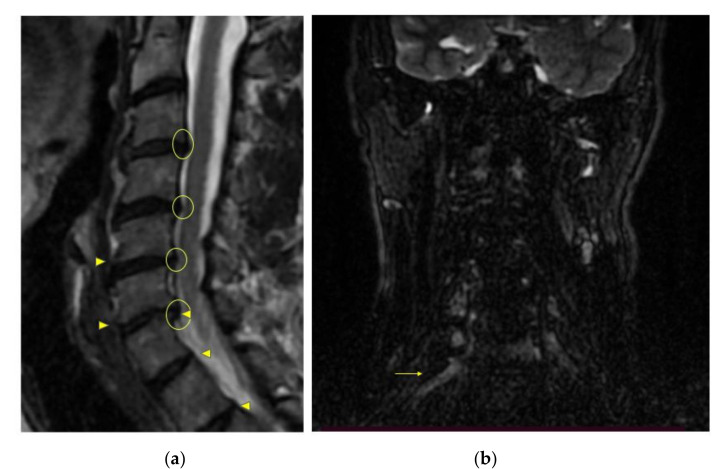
Cervical spine and Brachial Plexus MRI. (a) Sagittal T2 weighted image of the cervical spine. Straightening of the lordosis with spondylodiscoartrosic changes in C3-T1 tract are shown. The most evident features are presence of enthesophytes (arrowheads) both anteriorly and posteriorly, where they cause a reduction in intervertebral space height and moderate bulging anulus imprinting CSF column (yellow circles). (b) Coronal STIR image of the cervical spine. Right-sided hyperintensity aspect of the inferior trunk and medial cord of brachial plexus (yellow arrow) at C8-T1 level, indicating edema at this level, corresponding to the ulnar nerve symptoms.
